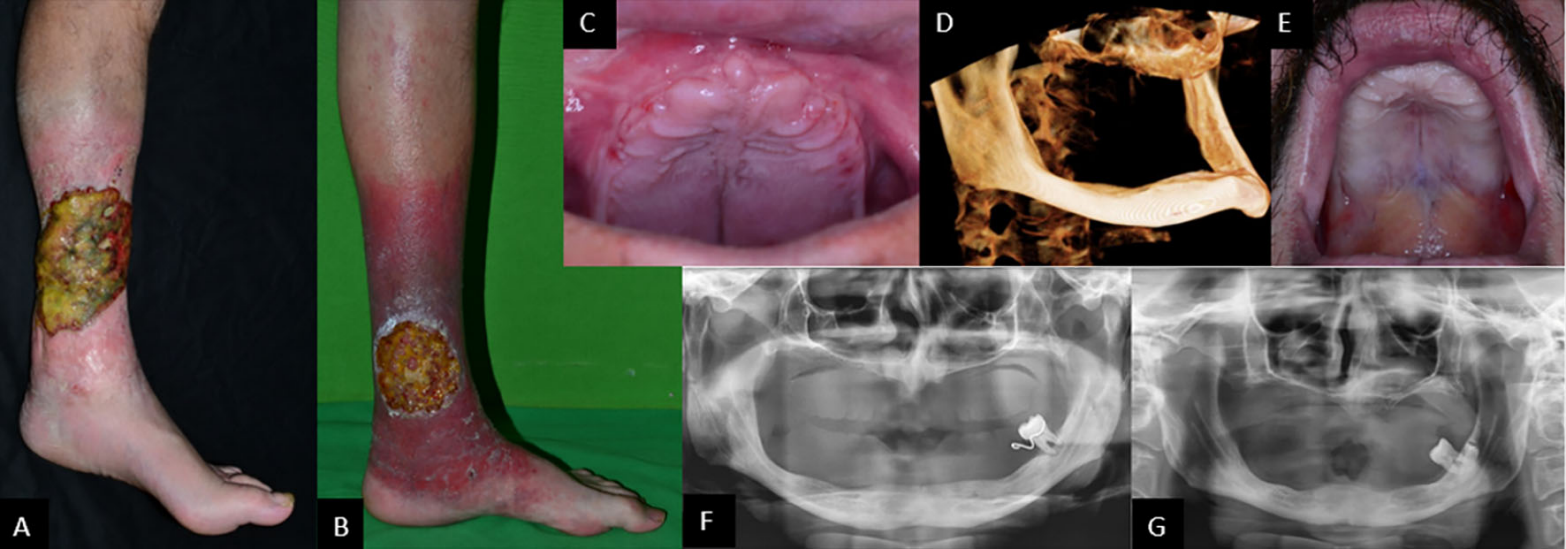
Biofilm-covered, 14-×-18-cm large ulcer in P1 (A) and 10-cm-diameter ulcer in P2 (B), both on left leg. Complete tooth loss in P1 (C) and P2 (E). Cone-beam CT scan showing severely atrophied jaws and missing processus alveolaris (D). Panoramic radiographs showing highly atrophied jaws and a single remaining erupted tooth in both patients: P1 (F) and P2 (G).
